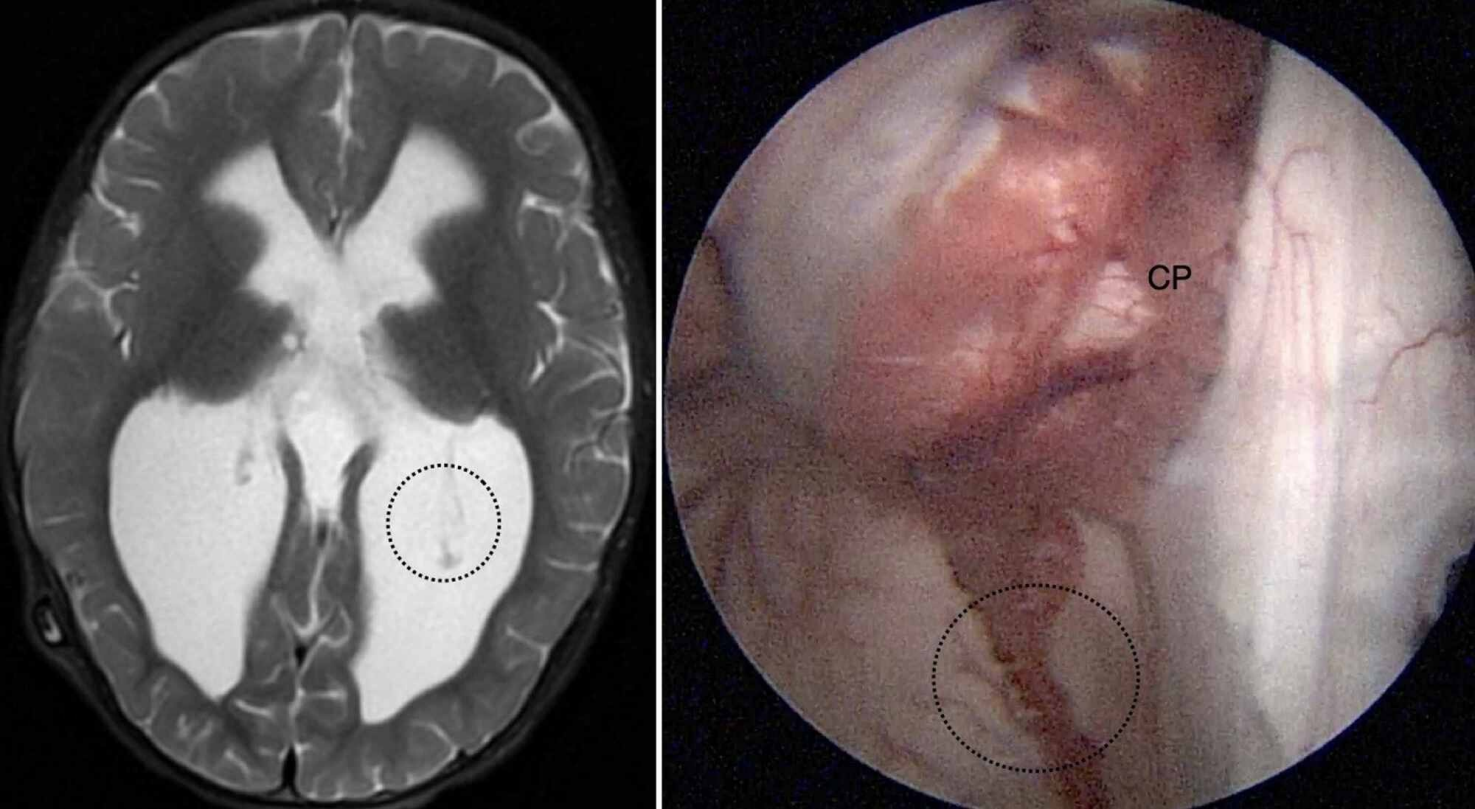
The type II anatomic variation of the glomus of choroid plexus (GCP). Type II GCP is classified as mobile, and the elongation is moderate. The glomus of left lateral ventricle reached the middle of distance between the fixation and the posterior limit of the occipital horn, as observed in an axial view on T2-weighted magnetic resonance imaging (left). In this endoscopic view of the left lateral ventricle, the GCP (indicated by the black dotted circle) is mobile and suitable for resection by our technique. CP, choroid plexus.
